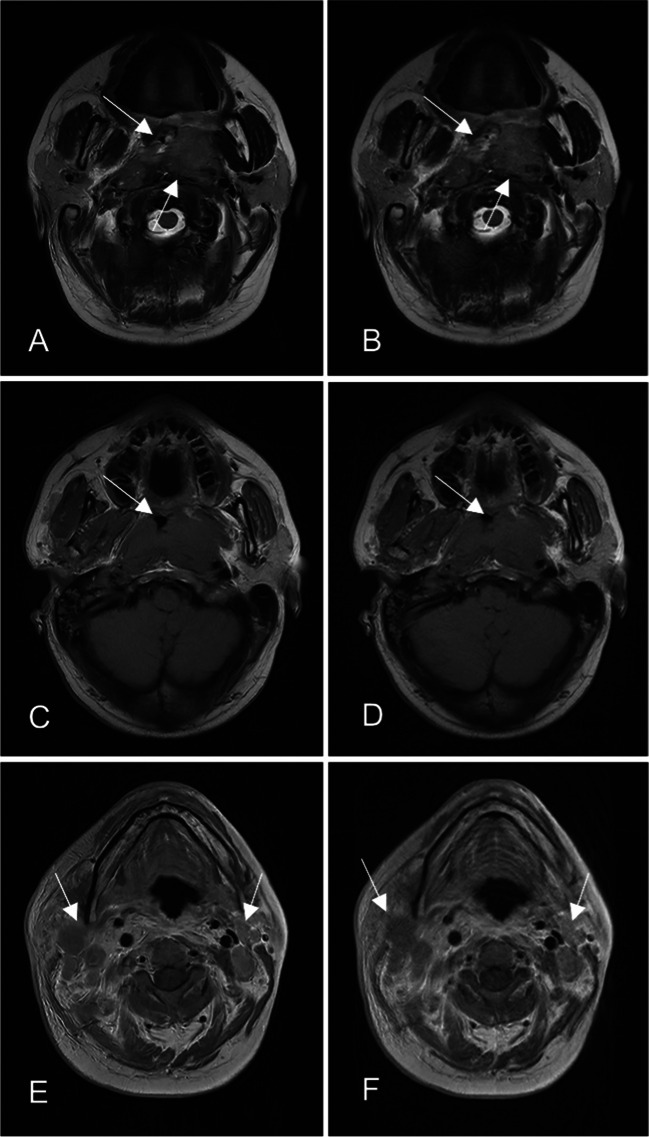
A 34-year-old male patient with NPC. The MRI sequences and examination time were summarized as follows: T2-weighted FSE sequences in the transverse planes: (A) with ACS technique (39.6 s); (B) with PI technique (59.0 s); T1-weighted FSE sequences in the transverse planes: (C) with ACS technique (74.8 s); (D) with PI technique (105.4 s); post-contrast T1-weighted FSE sequences in the transverse plane: (E) with ACS technique (92.1 s); (F) with PI technique (129.9 s). The border of NPC was clearer for FSE sequences with ACS (A, C arrows) than for FSE sequences with PI (B, D arrows); the scan time was shorter with ACS (E) than with PI (F), and the artifacts of swallowing motion could be effectively suppressed (E, F arrows) due to shortening of time
